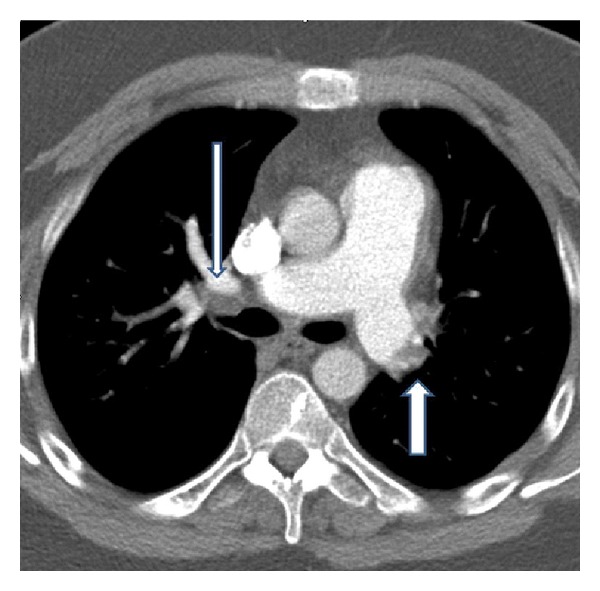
Computed tomography angiogram showing chronic thromboembolic clots in the central left and right pulmonary arteries (arrows).
